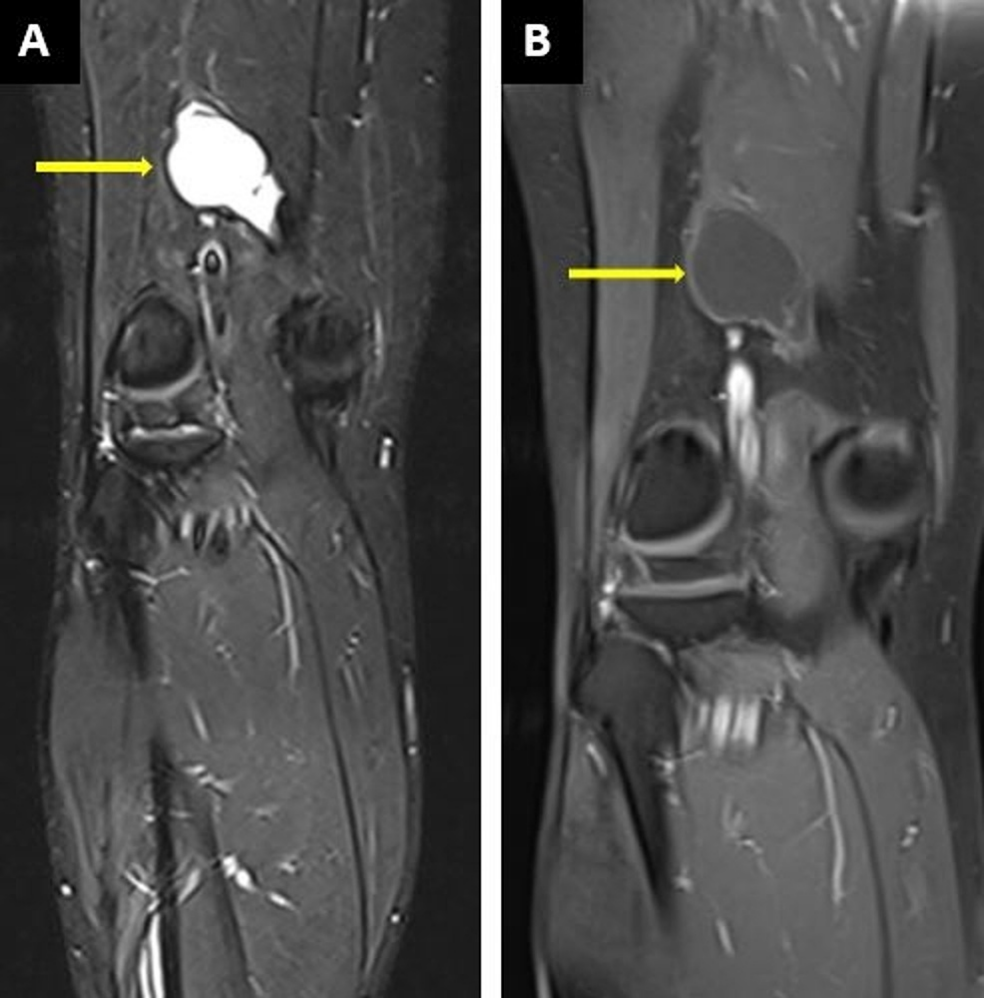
MRI of the right thigh (A) Coronal-STIR sequence demonstrating a well-defined high signal intensity lesion originating from the semimembranosus tendon. (B) On the post-contrast sequence, there is minimal rim enhancement with no remarkable internal enhancement of the lesion.
